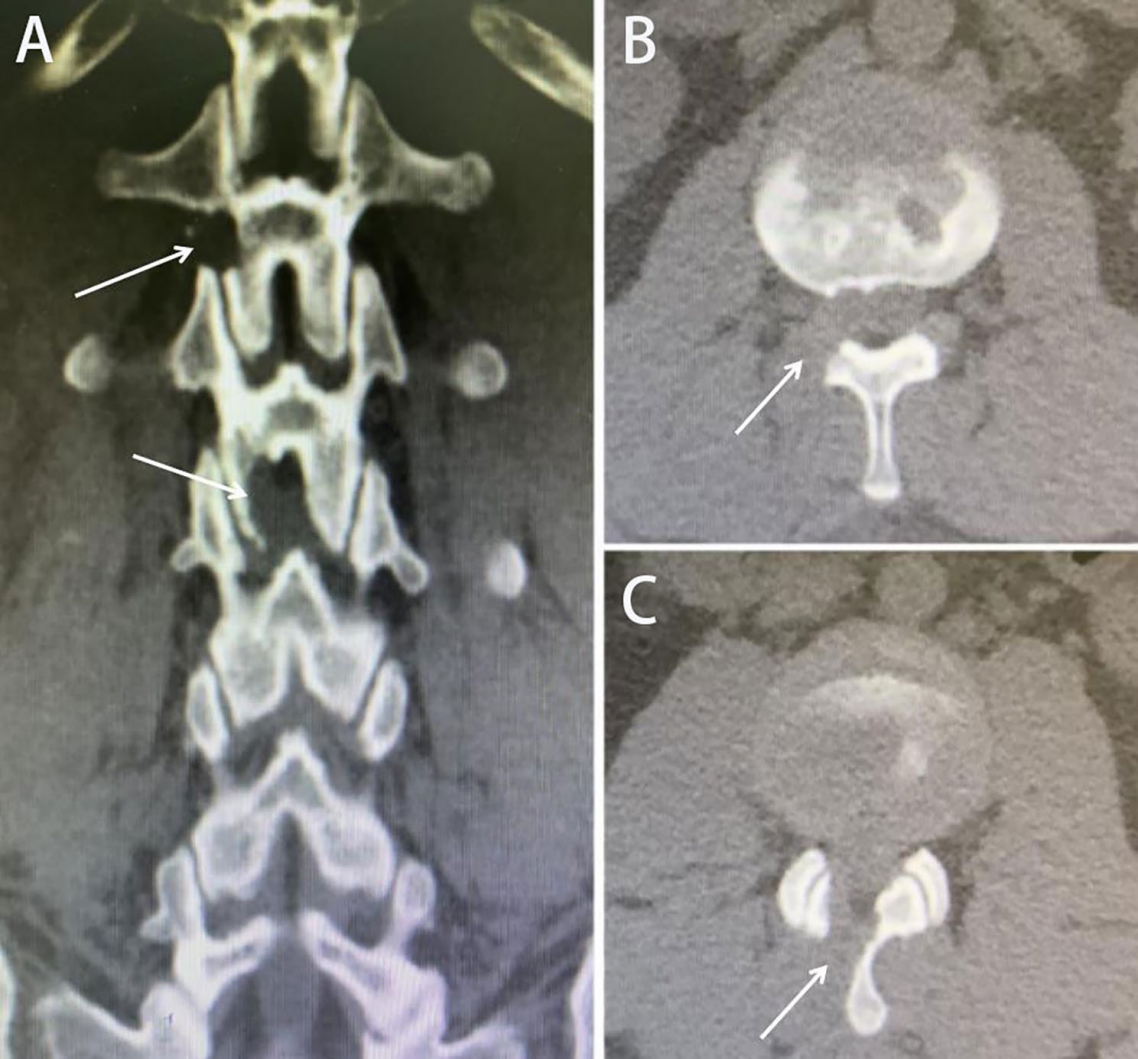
Postsurgical computed tomography (CT) showing the resection range of bone tissue in L1-L2 (paraspinal approach) and L2-L3 (interlaminar approach) (the white arrow). (A) Coronal view, (B) L1-L2, and (C) L2-L3.
